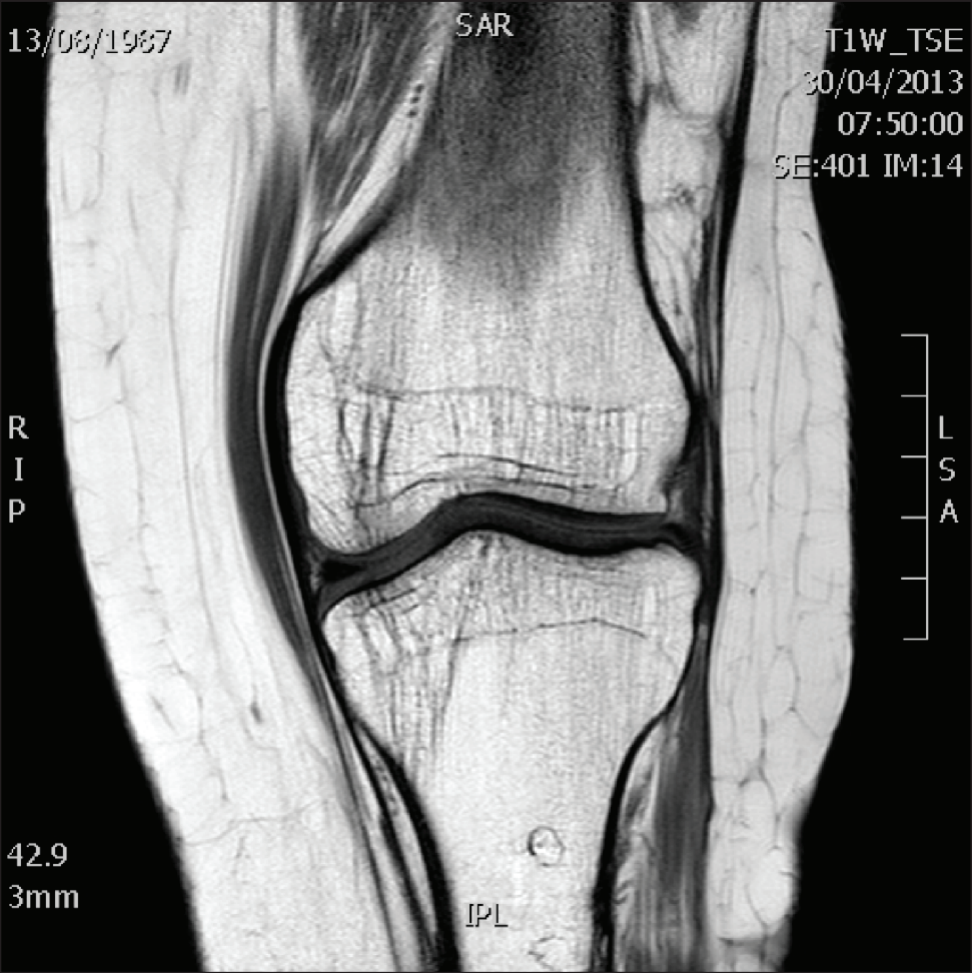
Coronal T1-weighted image of the left knee shows the shallow femoral notch and aplastic lateral tibial spine. The medial tibial spine is present but hypoplastic, also referred to as the “dromedar” sign (arrow). The anterior nor posterior cruciate ligament can be seen in the femoral notch.
